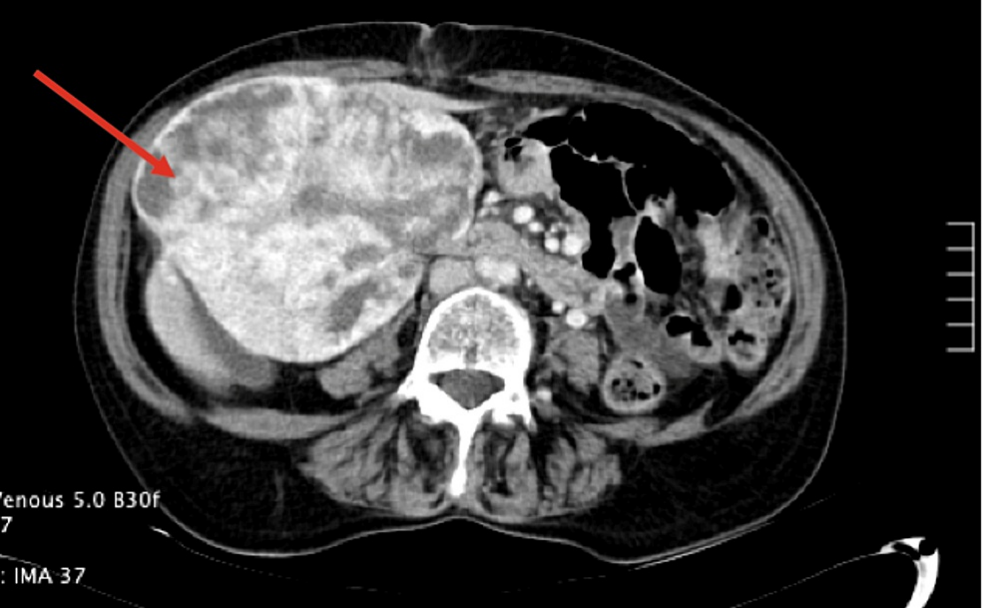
CT scan showing interval stability of the large liver lesion Lesion denoted with the arrow arising from the central liver. Her prior study had described an arterially enhancing liver mass with internal areas of necrosis in the right lobe of the liver consistent with a presumed focal nodular hyperplasia (FNH) with malignant degeneration.
